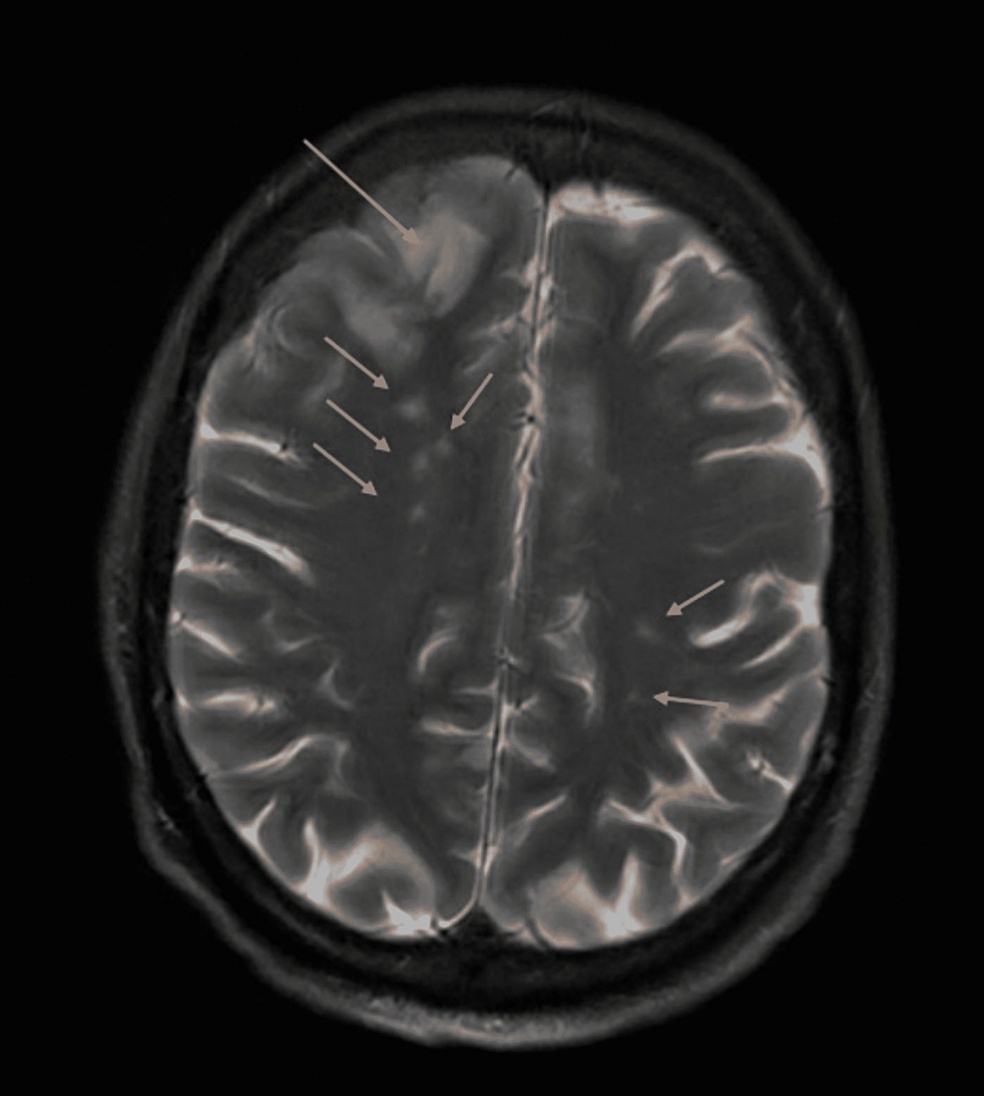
T2 MRI of the brain demonstrating multiple punctate foci in the bilateral frontal and parietal lobes consistent with embolic infarcts as well as subarachnoid hemorrhage in the right frontal lobe
